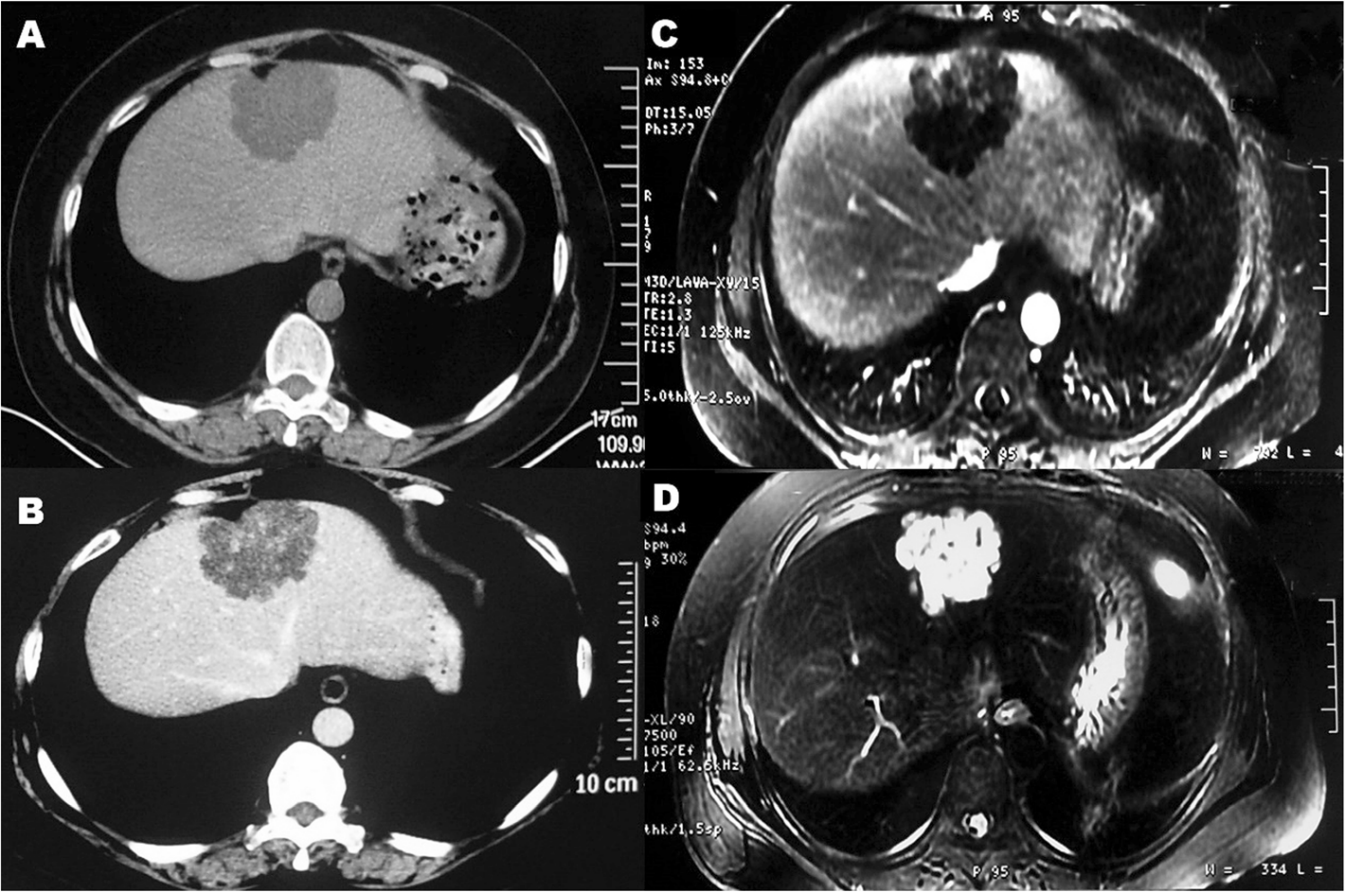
CT and MRI findings of liver mass in IVth segment. a plain CT scan. b Enhanced CT scan. c MRI-T1. d MRI-T2
